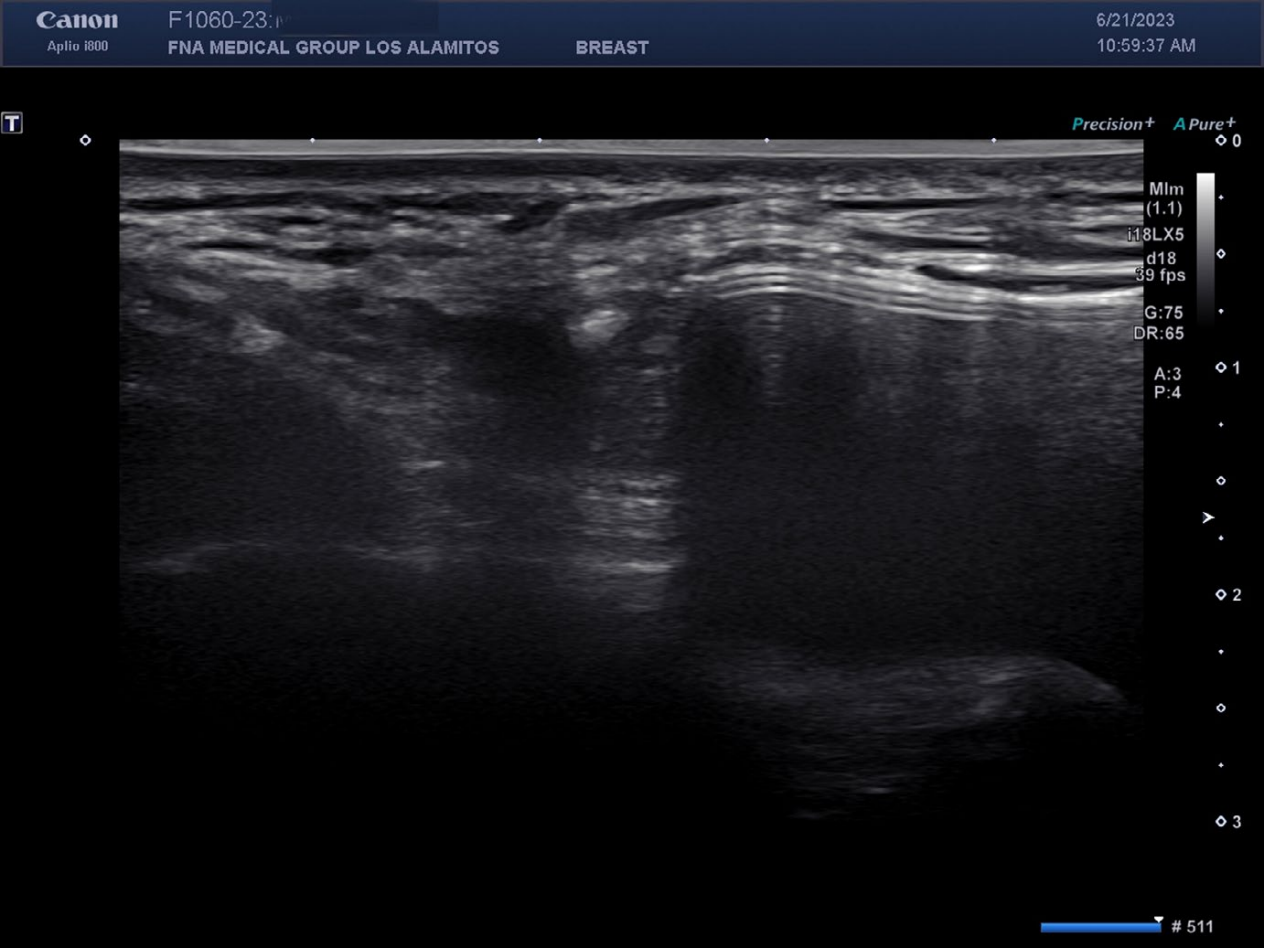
This patient has a silicone breast implant. It appears anechoic (black) on the right‐hand side of the screen. The pectoralis muscle and pleura at the left‐hand edge of the implant appear to suddenly drop deeper beneath the implant. Sound travels slower through silicone than through normal breast tissue. Therefore, the ultrasound machine assumes that the pectoralis muscle and pleura deep to the implant are much deeper than they really are. This speed propagation artefact results in a step‐off at the edge of the implant.
